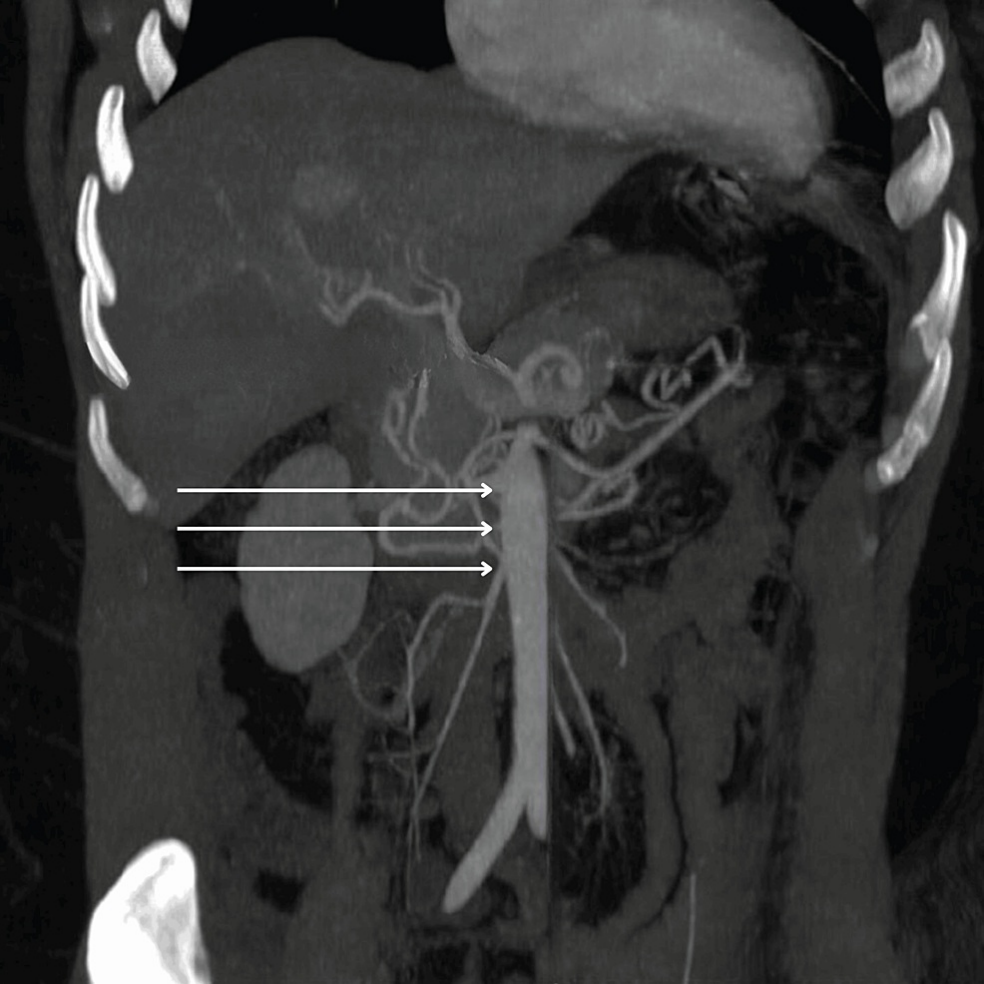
CT angiogram of the abdomen The image is depicting patent celiac, superior mesenteric, and inferior mesenteric arteries.
